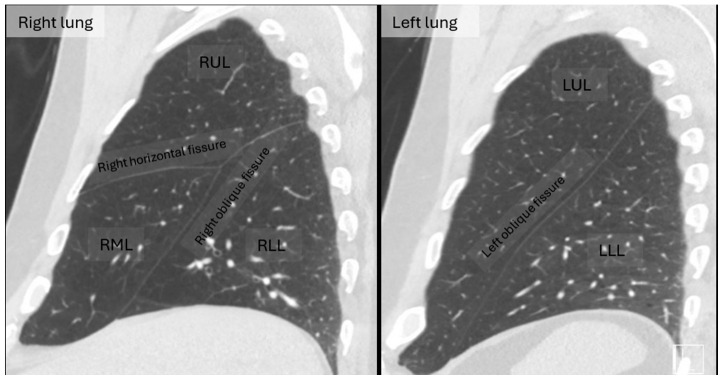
Chest computed tomography scans in sagittal plane demonstrating fissures assessed in the evaluation for endobronchial valve treatment. RUL: Right upper lobe. RML: Right middle lobe. RLL: Right lower lobe. LUL: Left upper lobe. LLL: Left lower lobe.
